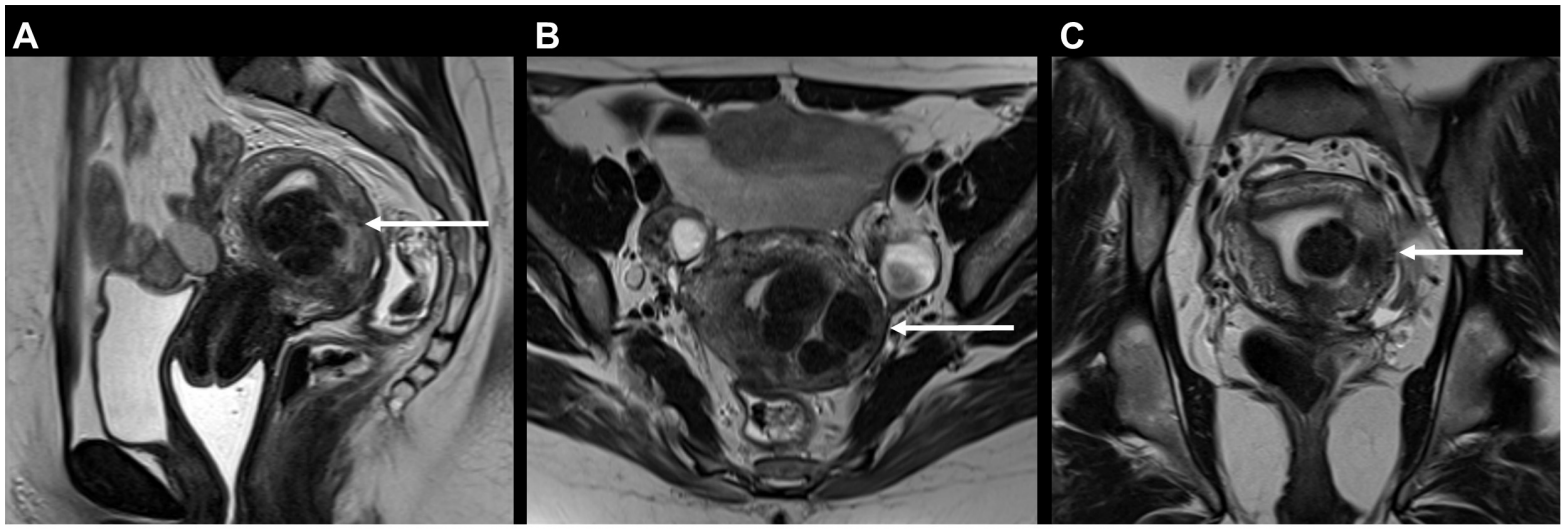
37-year-old patient with typical uterine leiomyomas (syn. fibroids) on MRI for endometriosis: (A) Sagittal, (B) axial, and (C) coronal T2 FSE (fast spin echo) showing mostly hypointense submucous and intramural masses of the uterine posterior wall measuring 3 cm in total (arrows). One submucous leiomyoma protrudes into the uterine cavity, largely surrounded by endometrium [type 1 according to the FIGO fibroid classification system (55)].
